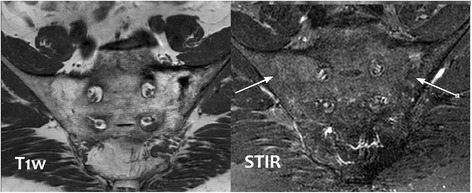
MRI of bony pelvis. The MRI of the patient’s pelvis reveals bony fusion of the bilateral sacroiliac joints with thickening of the cortex of the *left iliac bone* and bony trabeculation of the sacrum and *left iliac bone*. Degenerative changes are seen in the visualized *lower lumbar spine*. There is a hyperintense signal intensity in the *left sacral ala* which is likely due to micro trabecular insufficiency fractures
